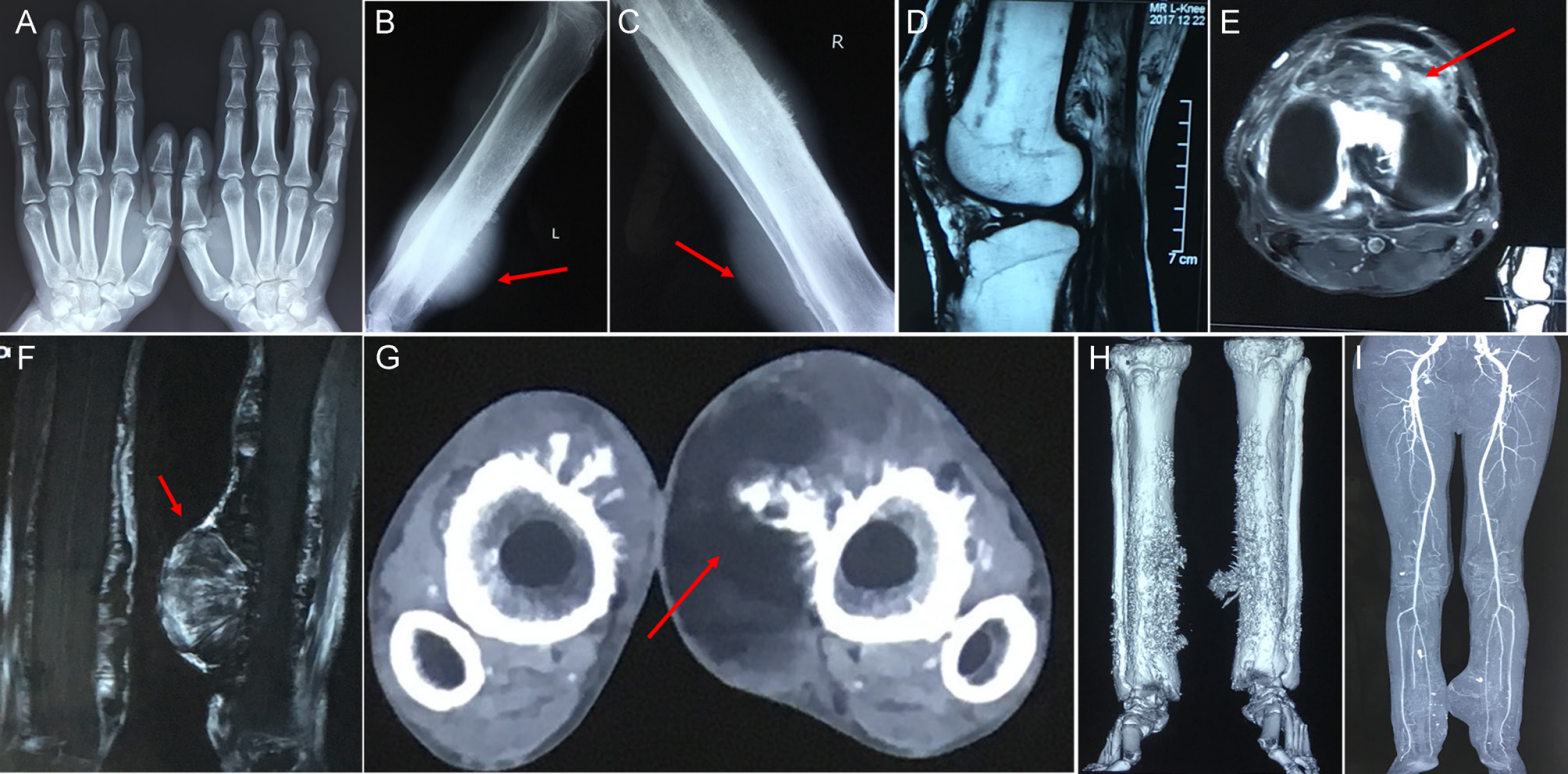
The radiographic signatures of the *HPGD*-mutated PHO patient. (A and C) X-ray examination of hands and bilateral knees. (A) Acro-osteolysis on distal phalanges of the fingers. (B and C) Cortical thickening and periostosis in the long bones, as well as the soft tissue swelling on the lower legs (red arrows). (D and E) MRI examination of bilateral legs. (D) Thickening of cortical bones and bone marrow edema in femurs and tibias. (E) Diffuse synovial hypertrophy and effusion in knee joint (red arrow). (F) An extra-articular diffuse tumor of the medialis of left tibia (6.2 × 11.2 × 10.6 cm) (red arrow). (G and H) CTA examination of bilateral legs. (G) Calcified soft tissue mass in the legs bilaterally, the biggest was in the left (11.3 × 5.5 cm) (red arrow). (I) Normal vascular perfusion of bilateral legs. And no increased vascularity was found inside the giant tumor.
